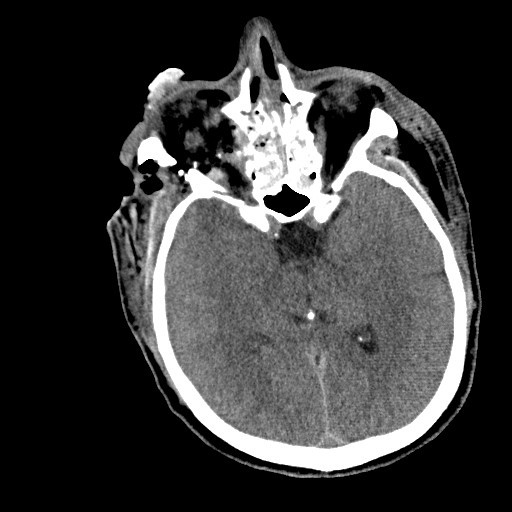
Right and left orbit extensive damage, CT cuts in sequential order.
